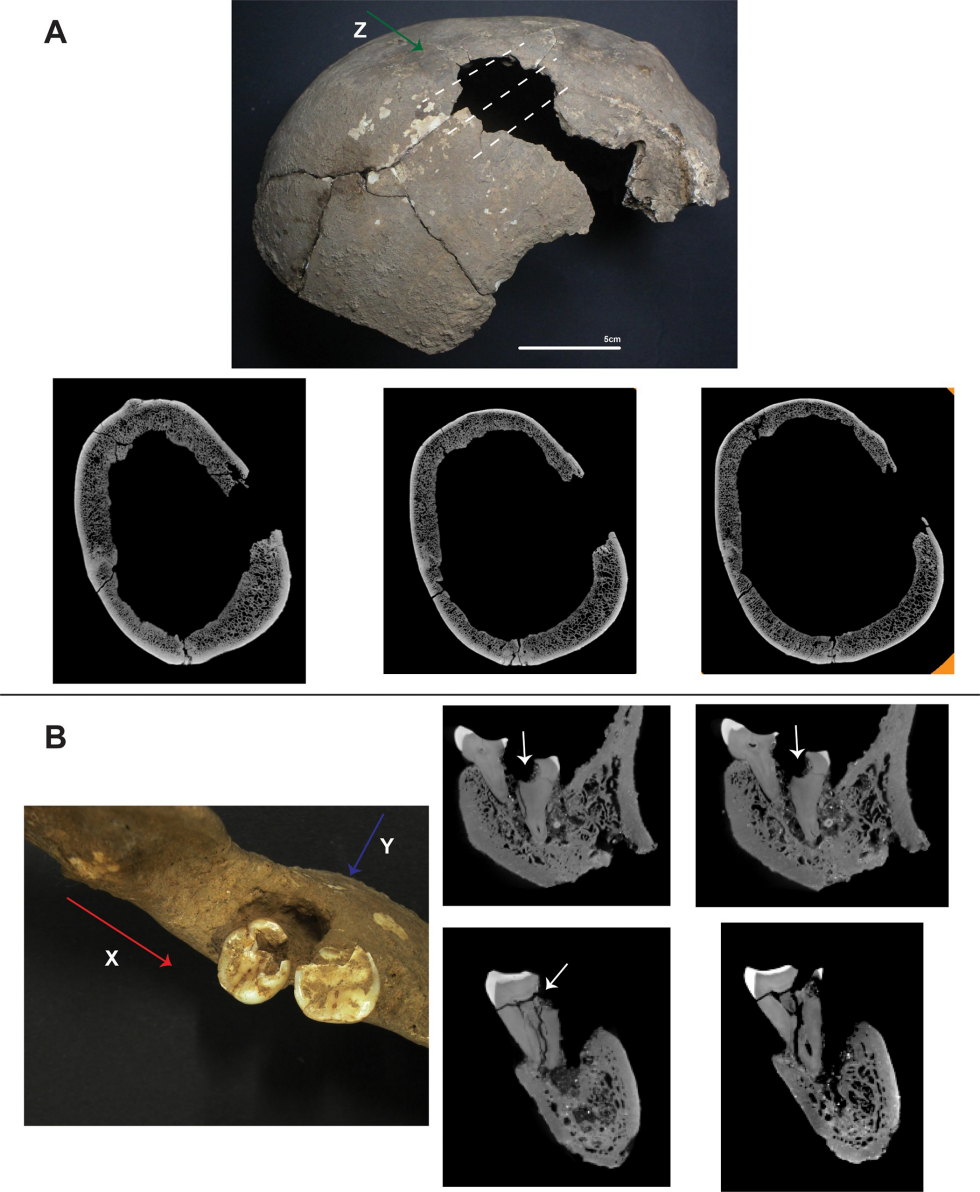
Micro CT-scans from TLB-1. A) Photograph of lesion on the right parietal (top) and CT images showing top (bottom left), middle (bottom centre) and bottom (bottom right) morphology of the lesion on the Z plane. Dashed lines indicate the location of the scanned images; B) Detail of dental lesion and CT images on the X (top) and Y (bottom) planes showing the morphology of the lesion (white arrows).
